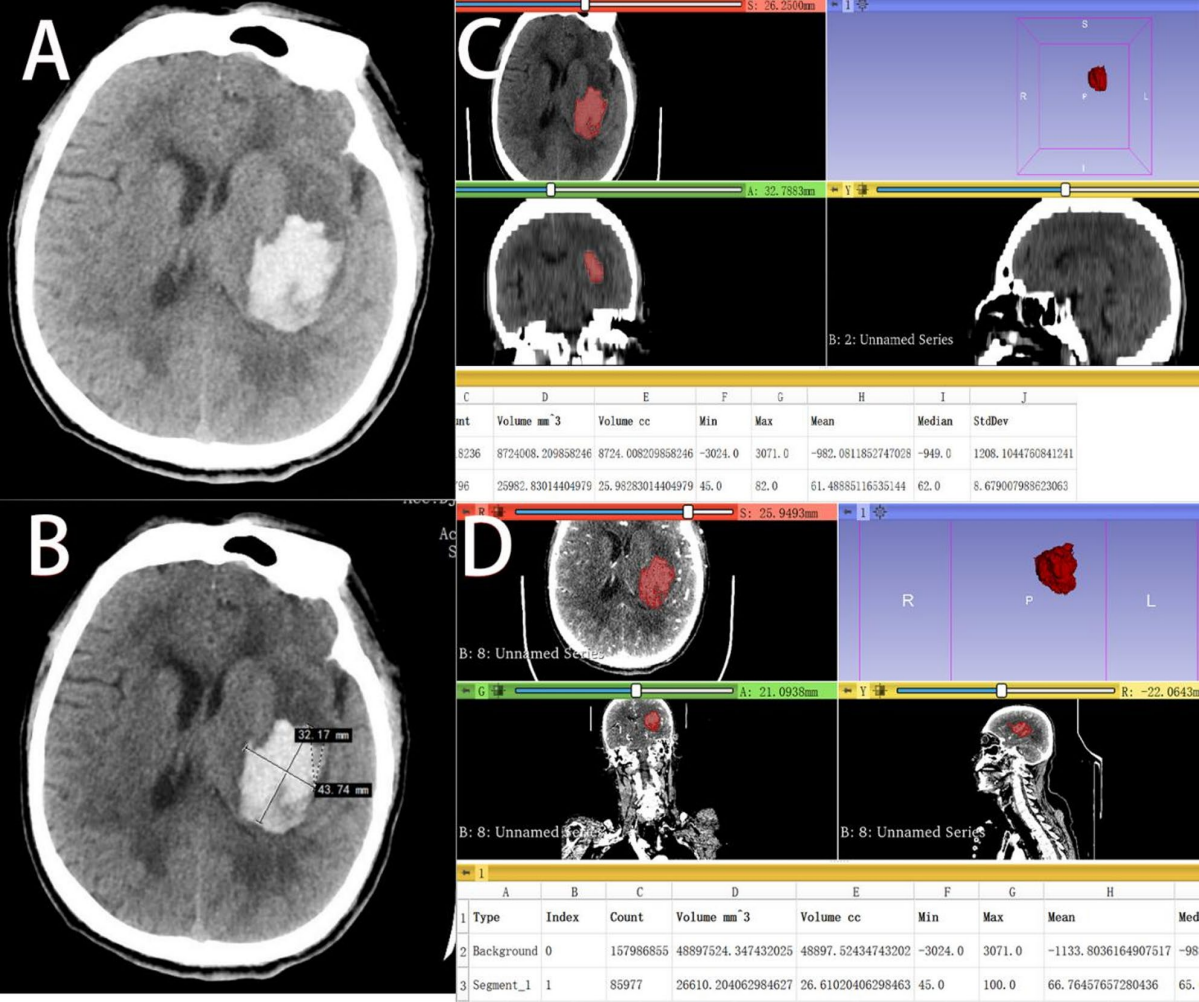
Comparison of Regular Hematoma Volume Calculations Using 3D Slicer and the Tada Formula. (A) Preoperative CT scan of the patient, showing the initial hematoma. (B) Preoperative hematoma volume calculated using the Tada formula (Tada-V), resulting in a volume of 24.6 mL. (C) Preoperative hematoma volume measured using 3D Slicer(3DS-V), resulting in a volume of 26.0 mL. (D) Preoperative hematoma volume was manually segmented on CTA source images(GSV), showing a volume of 26.6 mL
